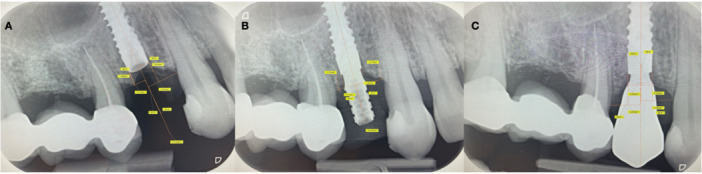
Radiographic bone level measurement for BLX implants. A line was drawn along the central axis of the implant, and a perpendicular reference line was drawn touching the coronal edge of the implant at the mesial and distal points; the vertical distances from this reference line to the bone and to the mesial and distal bone peaks were then measured. (A) Measurement of the implant without a cap screw. (B) Measurement of the implant with a temporary abutment. (C) Measurement of the implant with the final crown.
